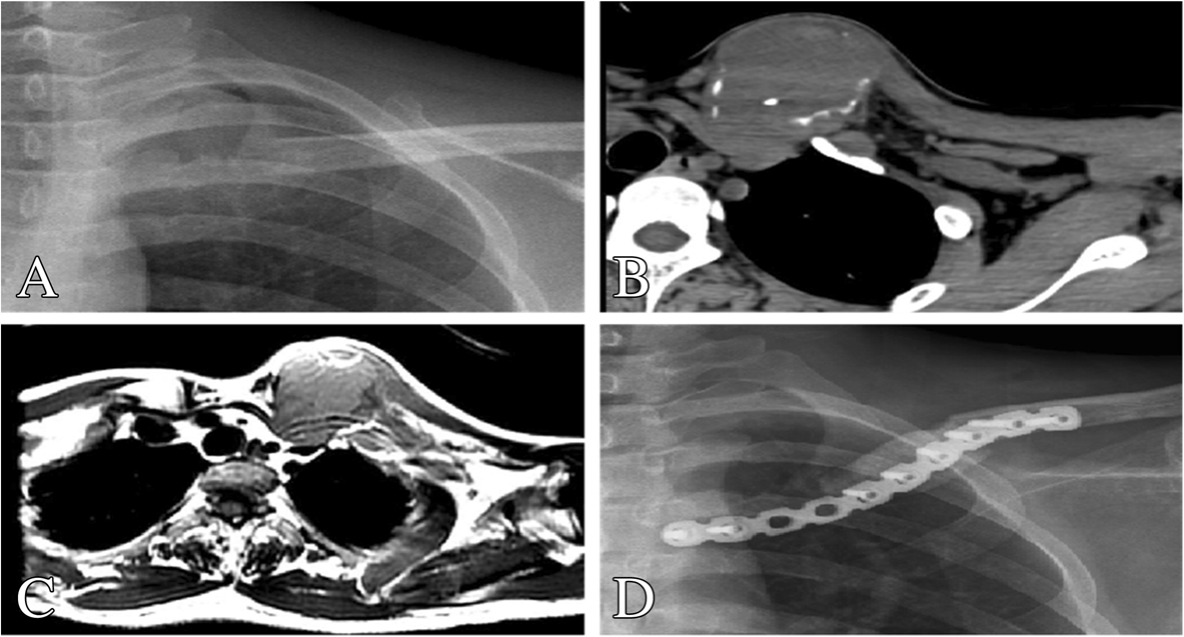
A 55-year-old man with left, lateral, and metastatic cancer. b Preoperative plain X-ray. b, c CT and MRI show an obvious tumor destruction on the medial left clavicle. d Postoperative X-ray 1 year after surgery
